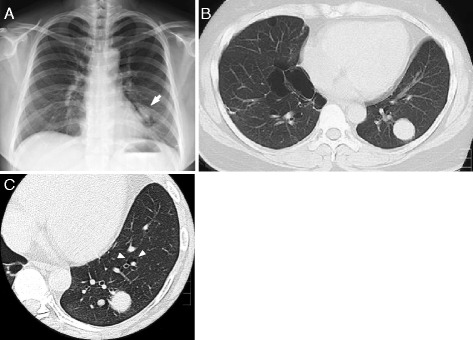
Plain radiograph and computed tomography of the chest. Plain chest radiograph on admission showed a nodular shadow superimposed on the edge of a cardiac silhouette (a). Computed tomography of the chest demonstrated a round nodule (the larger nodule, 25 mm in diameter) with a clear margin in the lower, left lobe and multiple irregularly shaped cysts in the bilateral lower lobes (b). Another axial image showed a smaller nodule (5 mm in diameter) having a similar characteristics with the larger nodule (c). Note that a small cyst abutting a pulmonary artery (*white arrowheads*)
